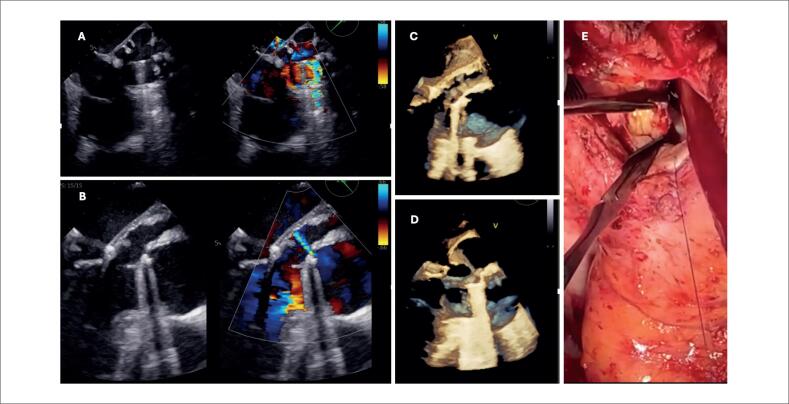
Ecocardiografia transesofágica e achados intraoperatórios. A - Vista em eixo curto da valva aórtica mostrando movimento de "balanço" da prótese, compatível com deiscência, e regurgitação periprotética grave entre as 7 e as 11 horas, envolvendo 25% da circunferência protética. B - Vista em eixo longo da aorta demonstrando um espaço perivalvar anecoico com fluxo Doppler colorido e expansão sistólica, compatível com um pseudoaneurisma periprotético (na fibrosa mitro-aórtica). C e D - Imagens 3D de ecocardiografia transesofágica delineando o pseudoaneurisma, estendendo-se cranialmente para a aorta ascendente e caudalmente para o ventrículo esquerdo. E - Imagem intraoperatória mostrando extenso desbridamento do trato de saída do ventrículo esquerdo.
